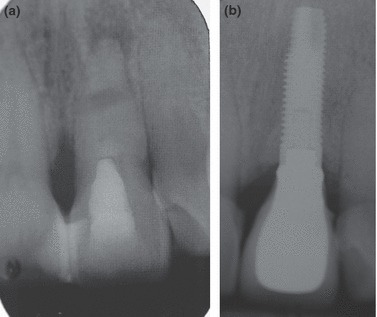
(a) Delayed presentation of a mid-root fracture and a periapical lesion. (b) The 21 was replaced with an implant.
